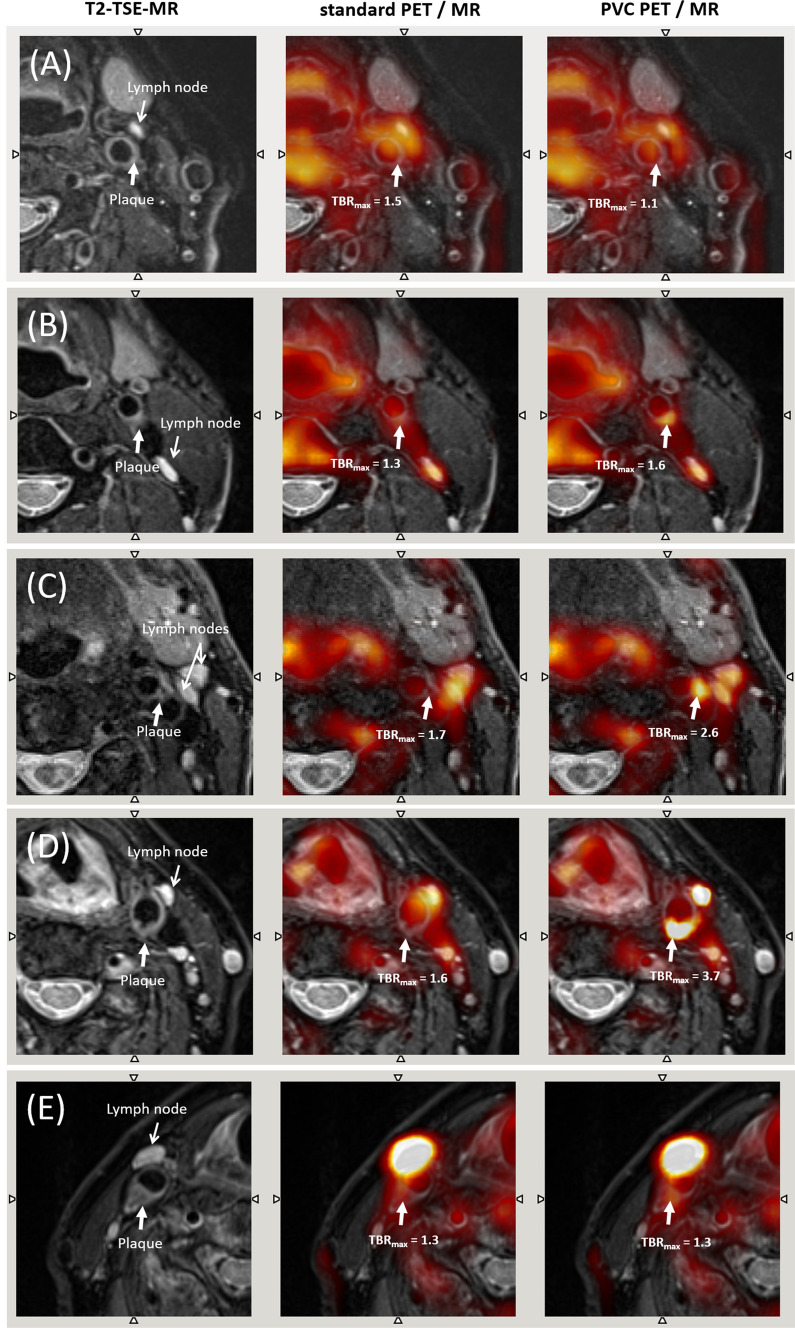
Axial MR (left) and PET/MR images before (centre) and after PVC (right) of different plaque types. From top to bottom (A-E) examples of plaques of type 1 to 5, respectively, are shown. (A) For plaque type 1, a small plaque with uniform morphology can be seen in the wall of the left carotid on T2-TSE MR. Unexpectedly for this type of plaque, a minor increase in uptake can be seen on the non-PVC-PET image (TBR = 1.5). However, when correcting the PVE, the TBR of the plaque is reduced to 1.1. This can be explained by the spill-in effects from a nearby hypermetabolic lymph node as seen on non-PVC already. As expected, for plaque types 2, 3, and 4 (B, C, and D), the PVC recovers the uptake in the plaque, thus, resulting in significantly increased TBR’s. (E) For plaque type 5, the PVC did not have a significant effect in the measured uptake of the plaque, due to the large size of the plaque. A small spill-in effect from a nearby lymph node is appreciated, but without an appreciable effect in the TBR values
